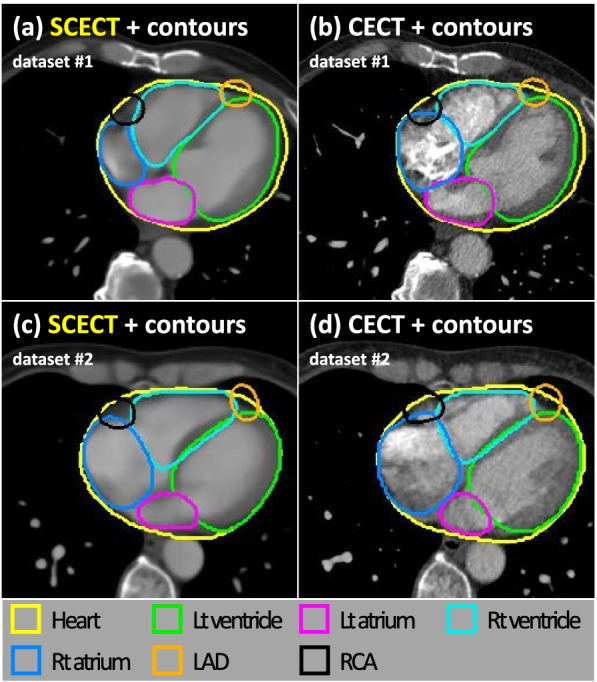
Representative results of manual contouring on synthetic contrast-enhanced CT (SCECT) (a, c) and contrast-enhanced CT (CECT) (b, d) images
